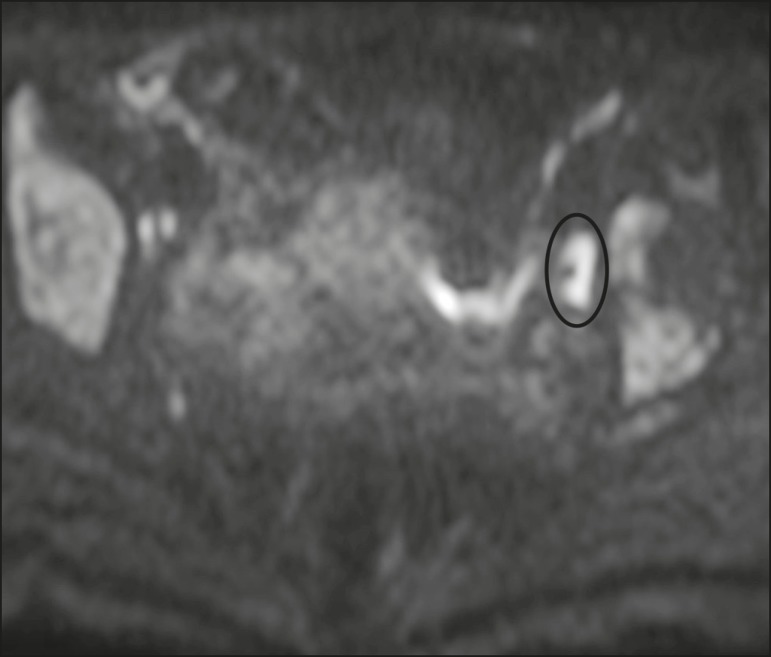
MRI of a pelvic lymph node in a female patient. Axial DWI showing a
